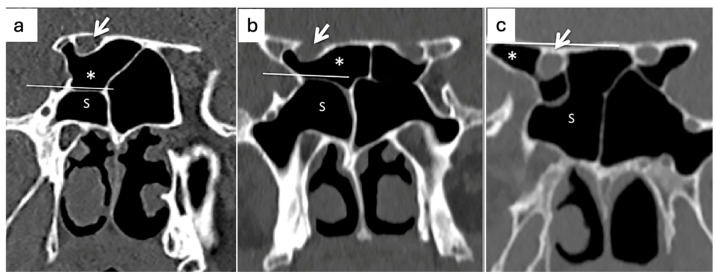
Coronal PNS CT scans. (a) Onodi cell (*) type I pattern of pneumatization is above and medial the horizontal line. (b) Onodi cell (*) type II is above and below the horizontal line. (c) Onodi cell (*) type III is below the horizontal line. A horizontal line was drawn at the uppermost part of sphenoid sinus (s) in the coronal image. Optic canals (white arrows) with bulging > 5 mm (type 4) are shown [30,31].
